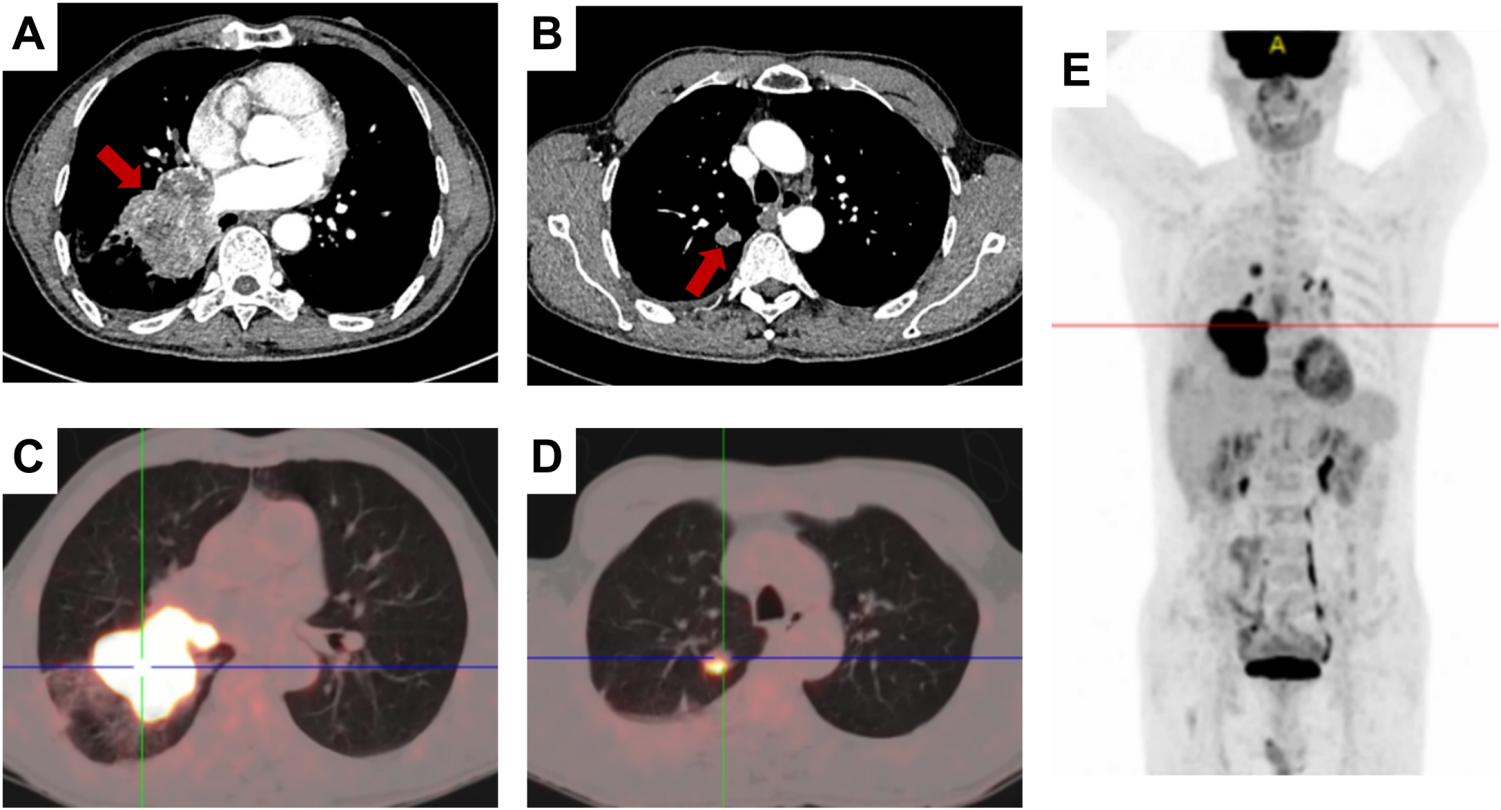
Radiological findings. (A, B) Contrast-enhanced chest CT showing a mass in the right lower lung lobe; the red arrow indicating the tumor region. (C–E) 18F-FDG PET/CT showing FDG avidity and extent of the pulmonary tumoral sites
